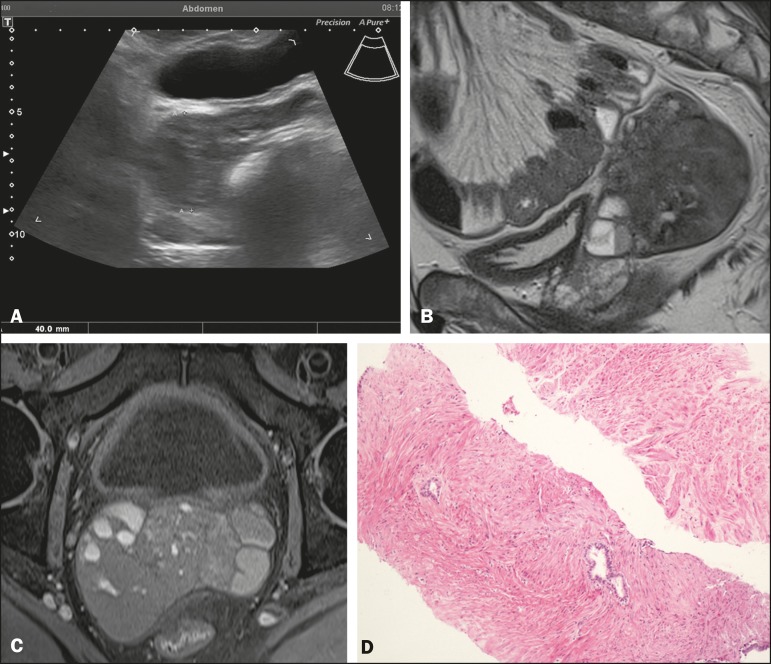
A: Transabdominal pelvic ultrasound showing a well-defined solid
